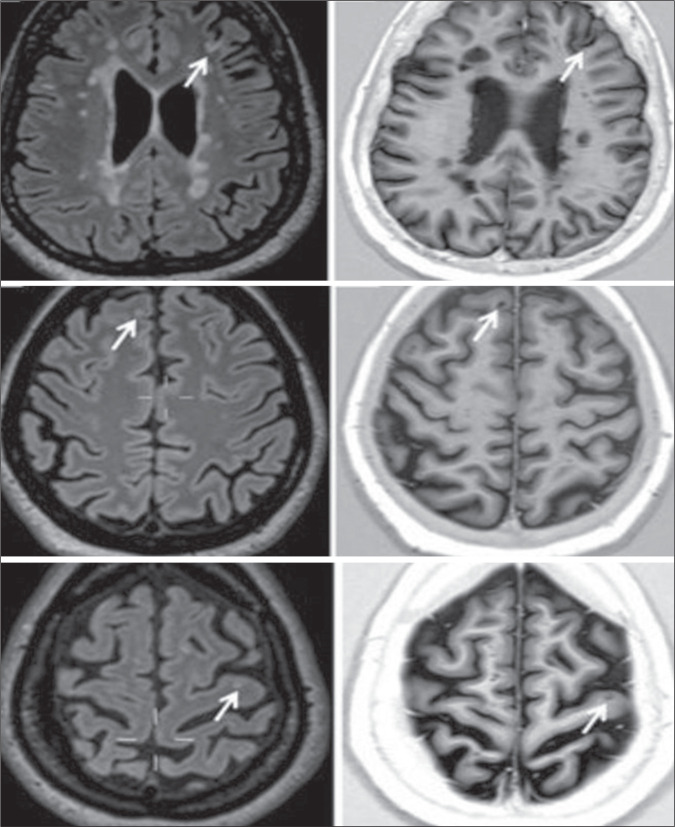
Axial 3D FLAIR (left) and axial PSIR (right): leukocortical (type I)
